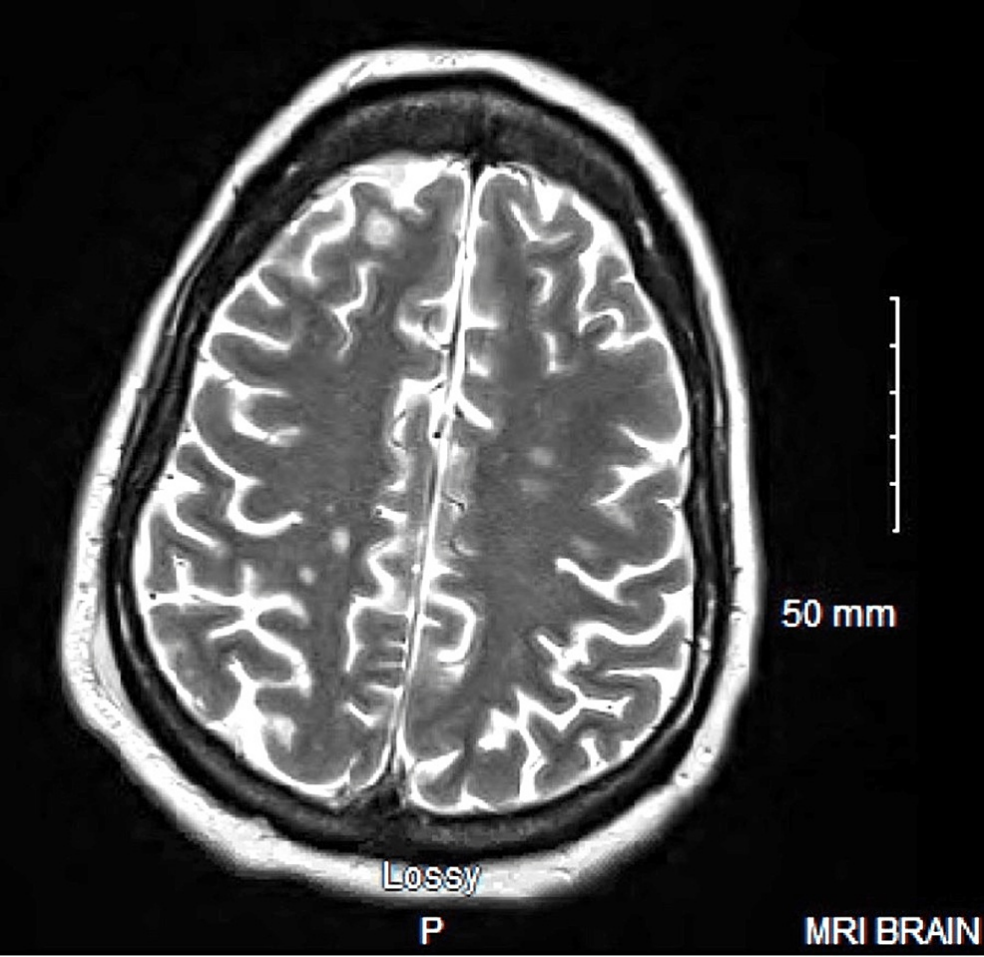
T2-weighted brain MRI The image demonstrates too-numerous-to-count areas of ring-enhancing lesions within the frontal, parietal, right occipital, brainstem, and cerebellar regions. Ring-enhancing mass seen within the right parietal scalp region concerning for abscess MRI: magnetic resonance imaging
